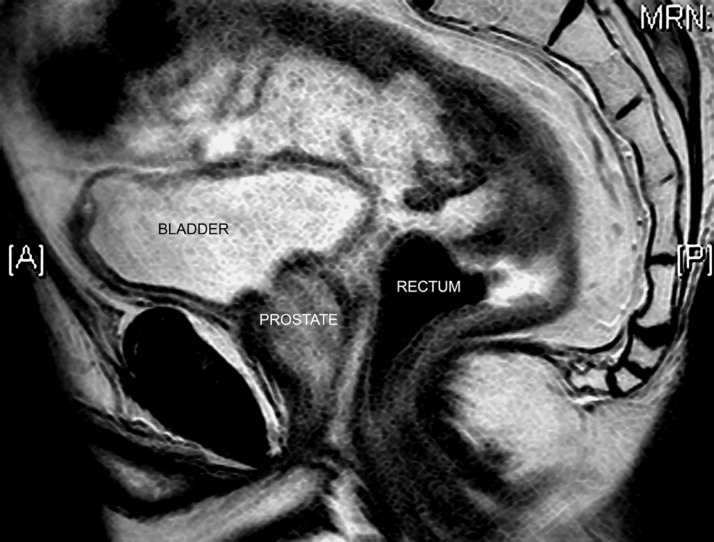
Midline sagittal pelvic magnetic resonance imaging demonstrating the proximity of the rectum and prostate
